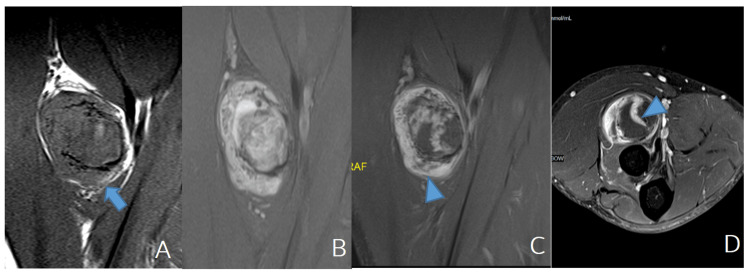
MRI imaging features: A-D: On MR, the mass is well-encapsulated with a surrounding ring of fat on T1 (arrow) (A). The lesion is primarily T1 hypointense and T2 heterogeneous (B) to hyperintense, with a fairly avid enhancement of the fibrotic capsular tissue component inside the mass on post-contrast imaging (arrowhead) (C, D). No bone marrow edema. A: MRI T1, coronal, the mass is well-encapsulated with a surrounding ring of fat on T1 (arrow); B: MRI T2, coronal; C: MRI T1 with contrast, coronal; D: MRI T1 with contrast, axial, hyperintense with fairly avid enhancement of fibrotic capsular of tissue component inside mass on post-contrast imaging (arrowhead).
